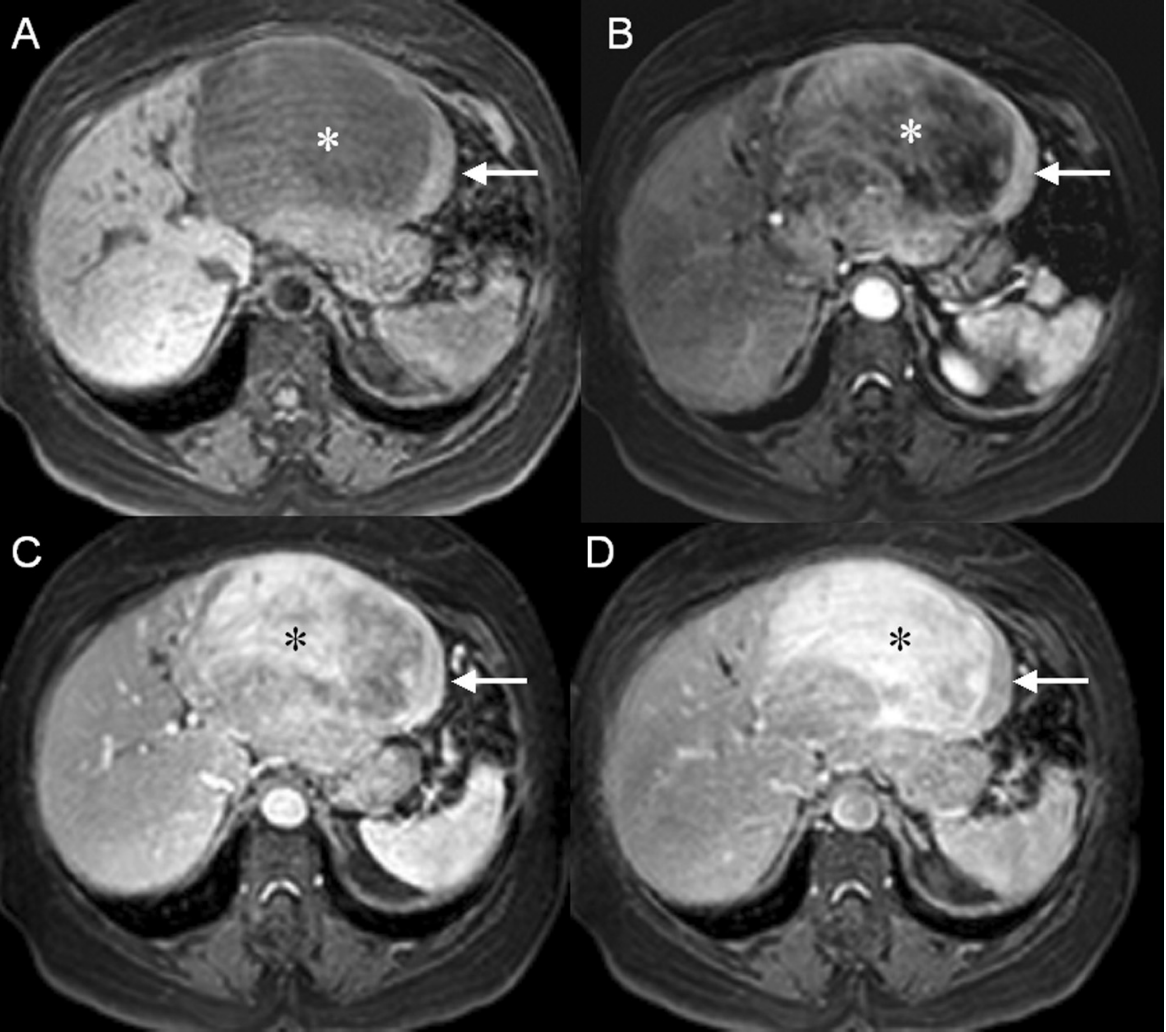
(A) T1-weighted fat-suppressed MR imaging without contrast; and MR imaging after contrast in (B) arterial phase, (C) portal phase, and (D) late phase. In T1-weighted images without contrast, the signal in the periphery of the mass (arrow) is similar to the muscle signal; the center of the lesion clearly shows a hypointense signal (*). Although impregnation of both components began in the arterial phase, the peripheral region (arrow) showed a stronger signal during the arterial and portal phases, followed by signal decay in the late phase due to relatively rapid washout, indicative of hypervascularization. The enhancement of the central component is far more intense in the late phase (*), probably due to a large interstitial space and slow flow through the vascular components.
